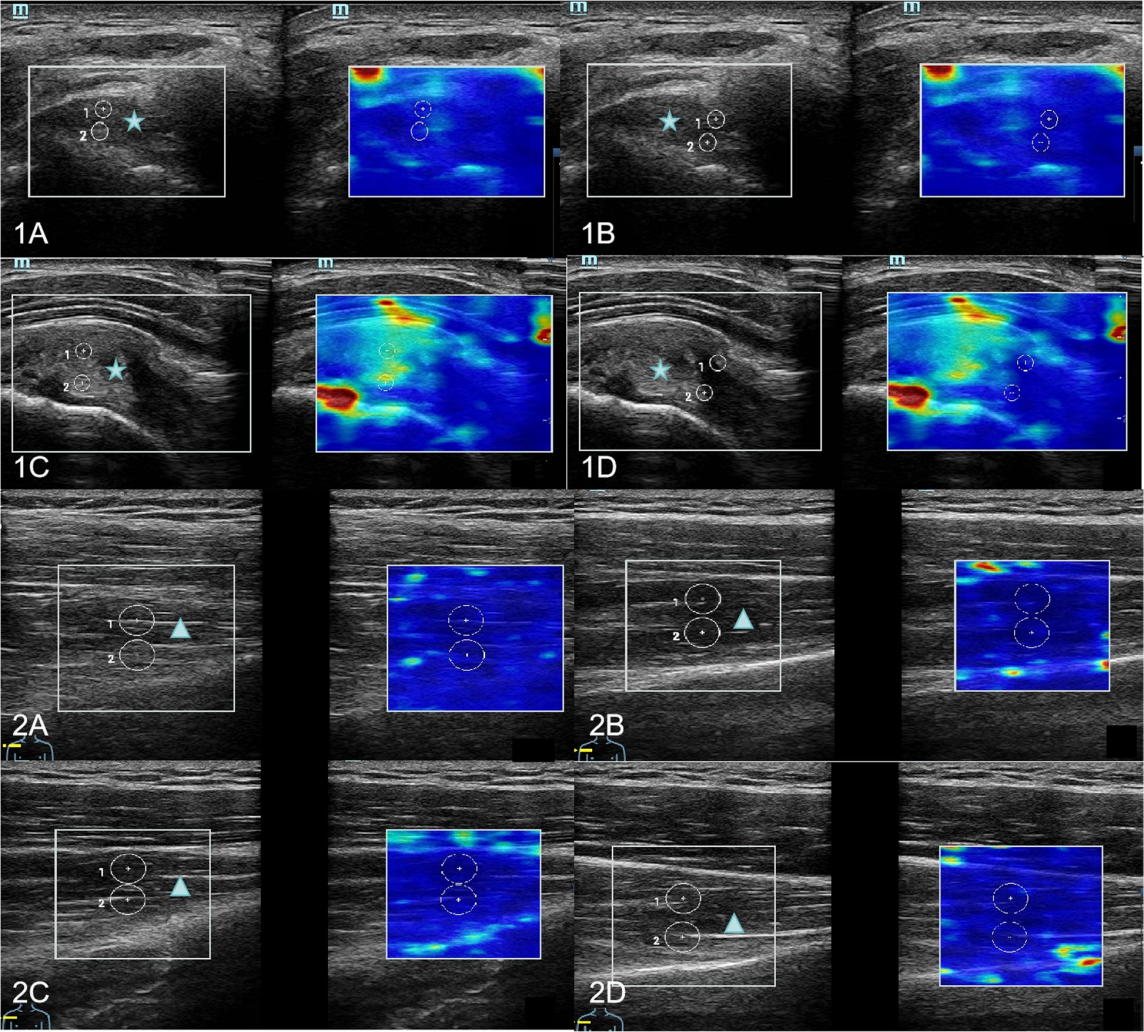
Ultrasound image of the supraspinatus tendon and muscle measured by SWE. A, B Natural position SWE measured SWV at four sites of the supraspinatus tendon; 1C-1D: modified crass position SWE measured SWV at four sites of the supraspinatus tendon. A, B Natural position SWE measured SWV at four sites of the supraspinatus muscle; 2C-2D: modified crass position SWE measured SWV at four sites of the supraspinatus muscle. Pentagram: residual tendon after tear of supraspinatus tendon. Triangle: supraspinatus muscle
